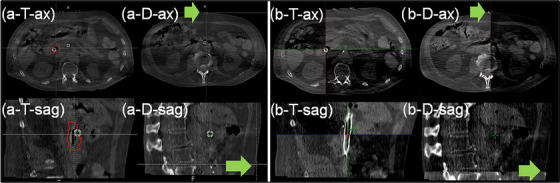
Tumor and donut‐shaped imaging‐surrogate identification on CBCTs. (a) positions of tumor centroids and donut‐shaped imaging‐surrogates were manually identified by Radiation Oncologists using the Ethos TPS, and (b) each CBCT (bright contrast) was registered to the fractional reference CBCT (darker contrast) by tumor and donut‐shaped imaging‐surrogate in ARIA. T: tumor, D: donut‐shaped imaging‐surrogate, ax: axial, sag: sagittal views. Red contour indicates the GTV. The green arrow points to the donut‐shaped imaging‐surrogate.
